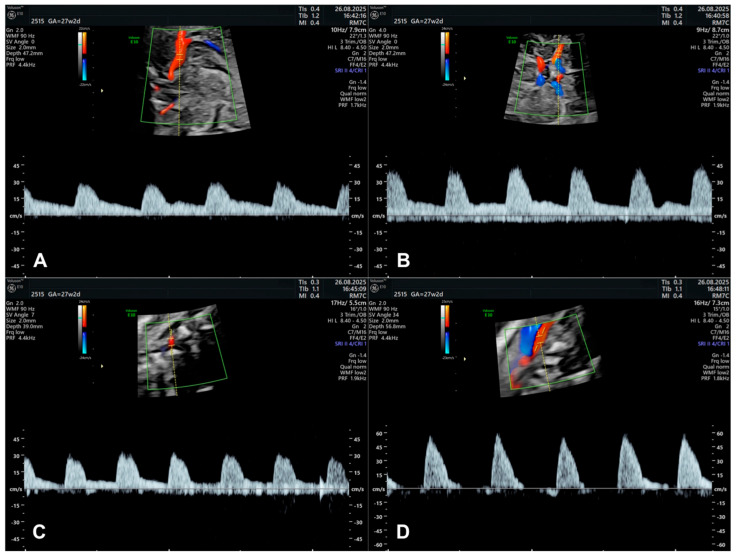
Normal velocity waveforms of different arterial territories at 27 weeks and 2 days of gestation (same patient), obtained by power Doppler evaluation recorded according to ISUOG Guidelines: Use of Doppler velocimetry in Obstetrics (2021). (A) Anterior cerebral artery (ACA): The waveform can be obtained in a sagittal plane and the vessel can be identified with color Doppler application, and should be obtained in the proximal segment of the artery. (B) Middle cerebral artery (MCA): The waveform can be obtained in an axial plane, in the proximal segment of the vessel. (C) Vertebral artery: Identified in a sagittal plane, running parallel to the spine. (D) Left internal carotid artery (ICA): Identified in paraxial plane, on each side of the neck, right below the jaw. Differences in maximum velocity in systole and diastole can be observed: internal carotid artery, more proximal to the heart, shows no diastolic flow and higher maximum velocity in systole; anterior and middle cerebral arteries show a positive diastolic flow with a lower maximum velocity in systole, findings associated with higher distance to the heart and intrinsic properties of these vessels. In pathologic conditions, autoregulation will accentuate those characteristics, reflecting the capability of decreasing resistance to blood flow.
